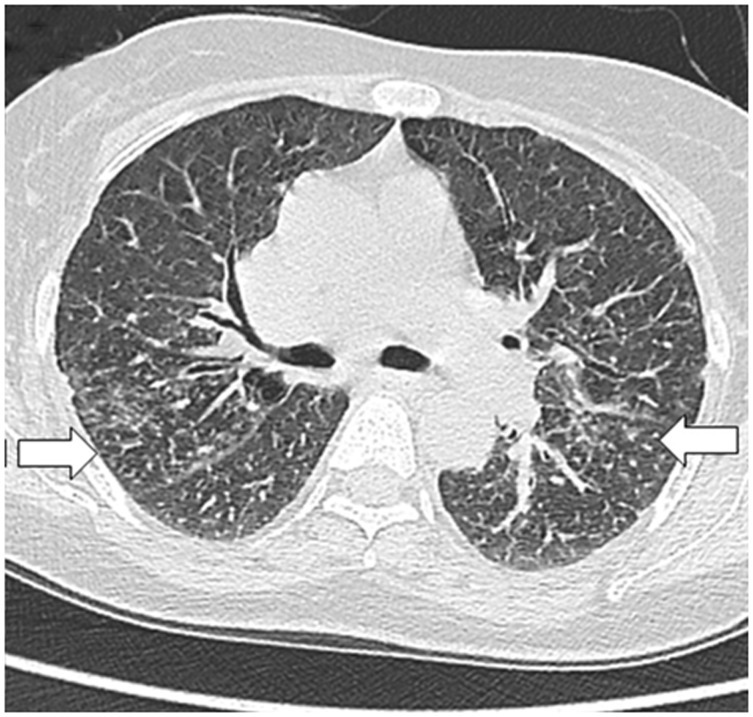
Sarcoidosis. High-resolution CT scan showing numerous tiny lung nodules with a perilymphatic and interlobular septal distribution (arrow).
